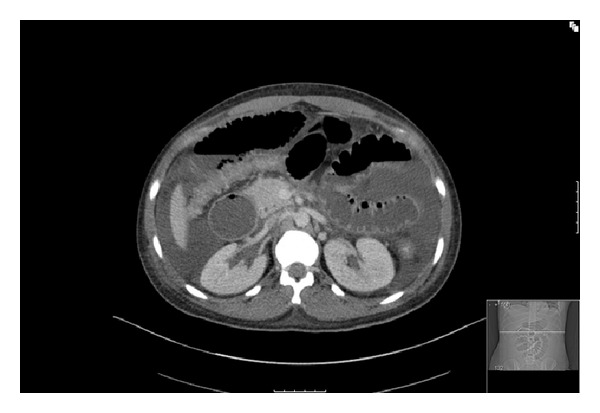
Repeat contrast enhanced CT of the abdomen and pelvis at day 3 after admission revealed pneumatosis involving the distal jejunum, proximal ileum, and diffusely dilated small bowel loops as well as segmented submucosal thickening of the small bowel.
